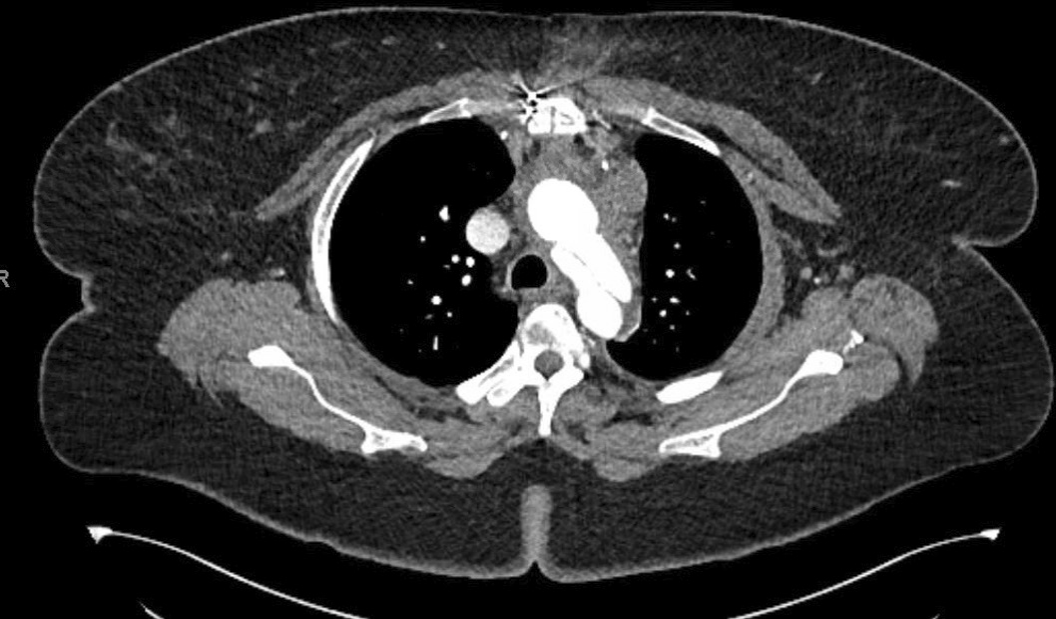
Postoperative computed tomography angiogram of the patient's repaired ascending aorta and proximal hemiarch, obtained 1 month after surgical repair of type A aortic dissection. The remaining, unrepaired flap distal to the repair can still be seen, also present in the descending and abdominal aorta.
